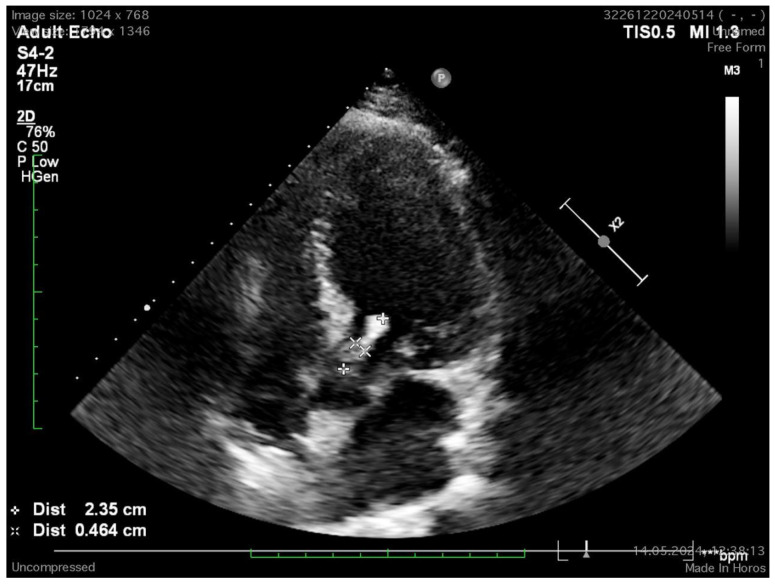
Representative echocardiographic image demonstrating valvular vegetation in infective endocarditis.
